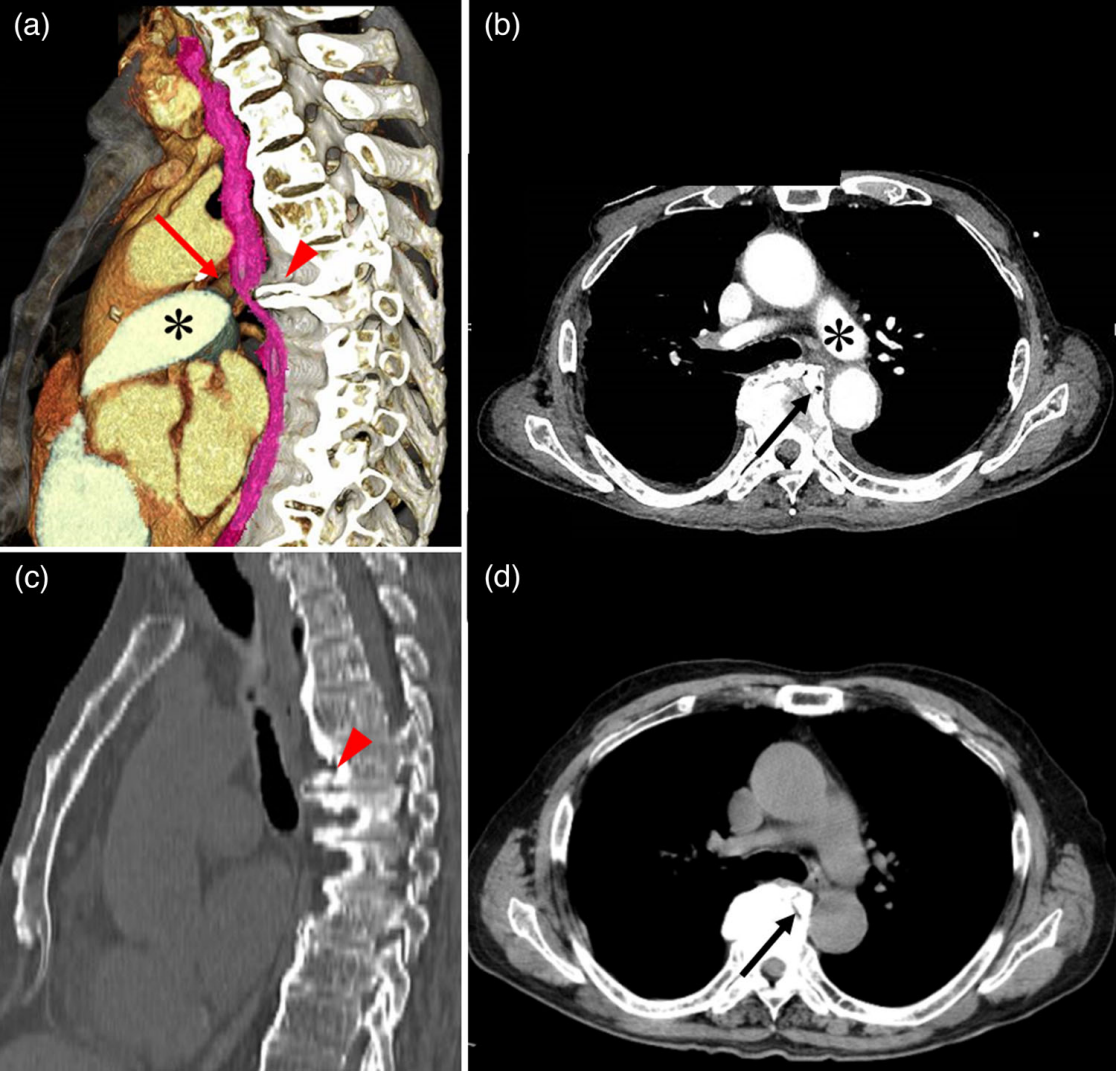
Computed tomography (CT) image on admission (a) Volume‐rendered, reconstructed sagittal section of the contrast‐enhanced CT image: The esophagus (red arrow) is constricted due to extrinsic compression from an osteophyte (red arrowhead) on the sixth thoracic vertebra, and the anterior esophageal surface is restricted by the left pulmonary artery (*). (b) Axial section of the contrast‐enhanced CT image: The esophagus has migrated to the left side of the trachea due to compression from the vertebral osteophyte (black arrow). The migration of its anterior surface is restricted by the left pulmonary artery (*). (c) Sagittal section of a plain CT image obtained at another hospital 8 years ago: The vertebral osteophyte (red arrowhead) appears smaller in size. (d) Axial section of the plain CT image obtained from another hospital 8 years ago: The vertebral osteophyte (black arrow) appears smaller in size.
